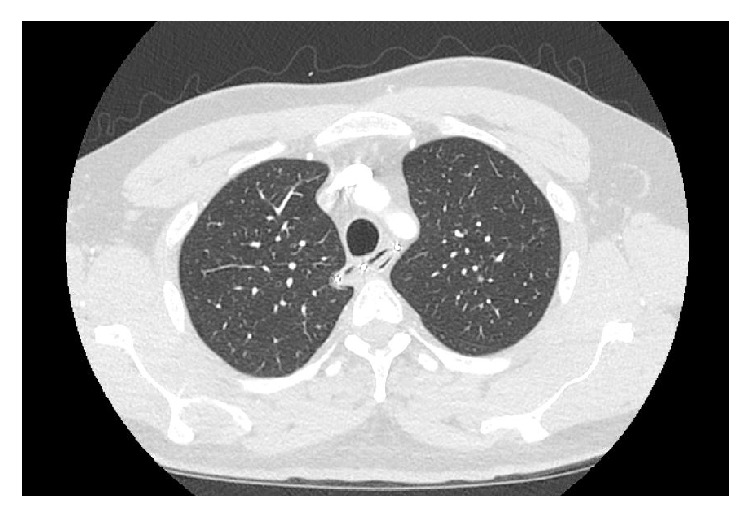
CT of the chest abdomen and pelvis showing metallic foreign body lodged in the upper esophagus without evidence of perforation.
